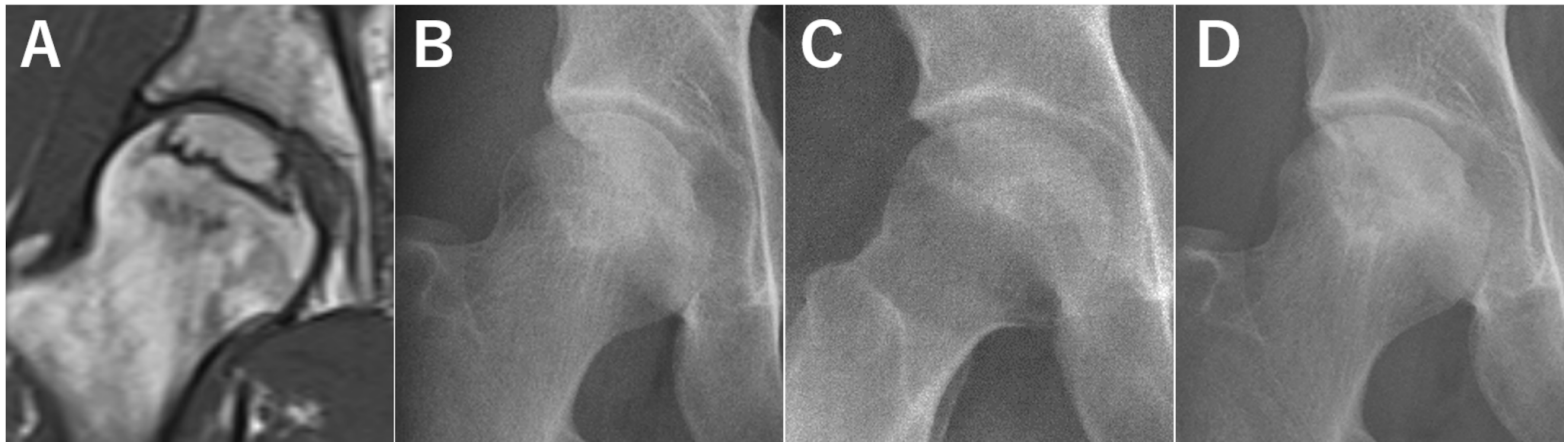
Osteonecrosis of femoral head of a 28-year-old man with discrepancy in the Japanese Investigation Committee type classification between radiography and magnetic resonance imaging (MRI). (A) The central coronal section of the femoral head on MRI T1-weighted image, it is classified as type C1. (B) The anteroposterior (AP) radiographic view of the femoral head, it is classified as type C2. (C) The lateral radiographic view. The extent of necrotic area is located anteriorly, and the extent of the AP necrotic region is 60%. (D) AP radiograph obtained 5 years after the first visit shows no collapse progression of the right femoral head
